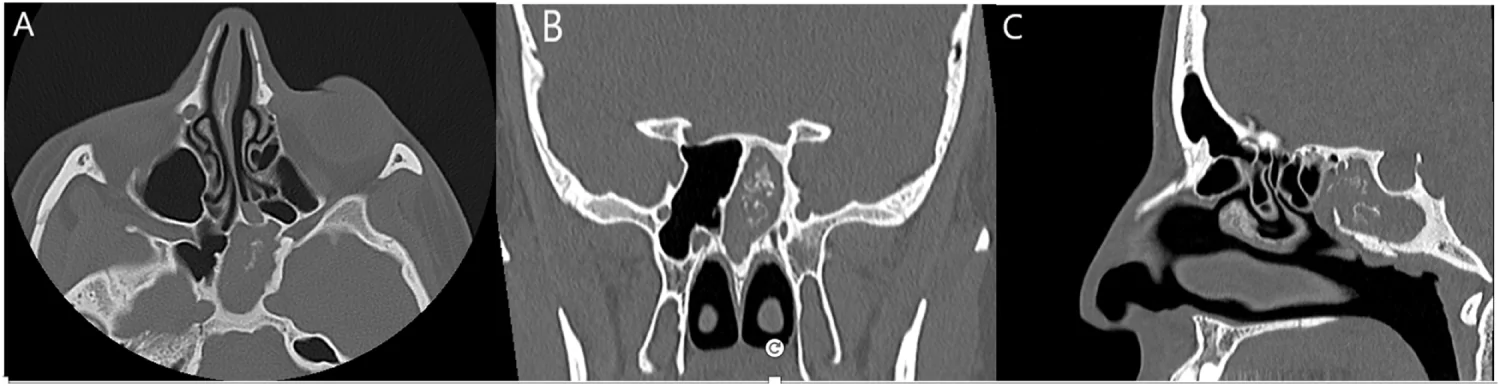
Non-enhanced CT scans of paranasal sinuses. (A) Axial view showing complete homogeneous opacification of the left sphenoid sinus. The contents show heterogeneous hyperdensity. (B) Coronal view showing wall thickening and sclerosis with central calcification of the left sphenoid sinus cavity. (C) Sagittal view showing left sphenoid sinus expansion with homogeneous opacification extending to the sphenoethmoidal recess and wall hyperostosis. There is no erosion into the sella, clivus, cavernous sinus region, or carotid canal.
